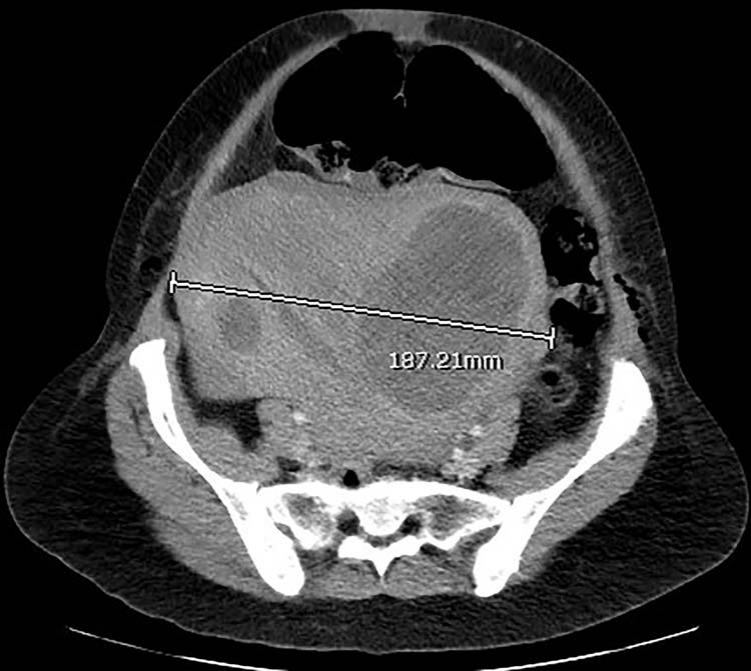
CT abdomen and pelvis with venous phase contrast, axial view. Polymyomatous uterus at its maximal width of 187.21 mm, 2 days postpartum. Note the larger fibroid on the right, reported to show necrotic changes. Dilated bowel loops visible anterior to the uterus.
